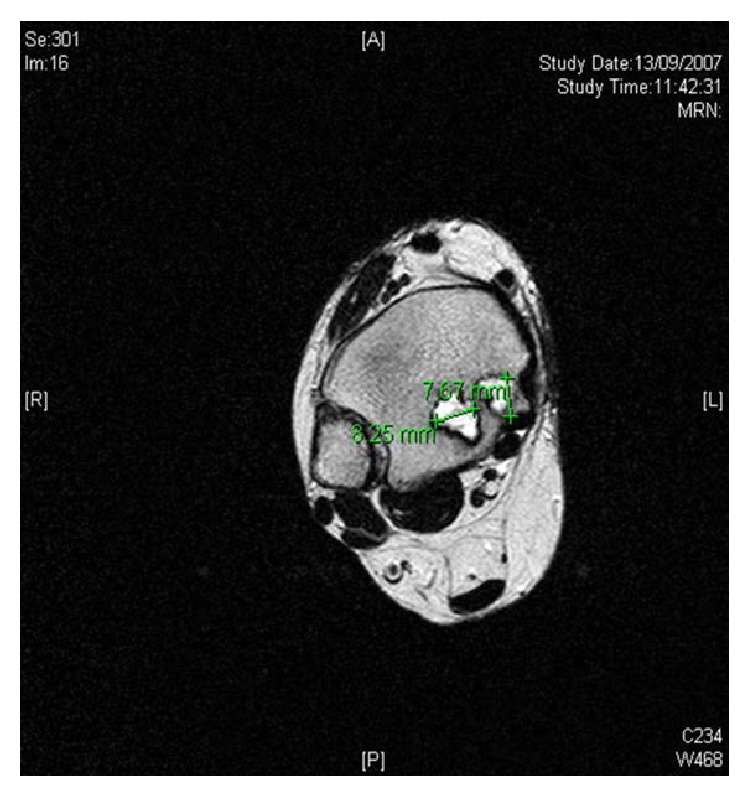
Axial view MRI showing well defined cystic fluid filled lesions in distal tibia.
